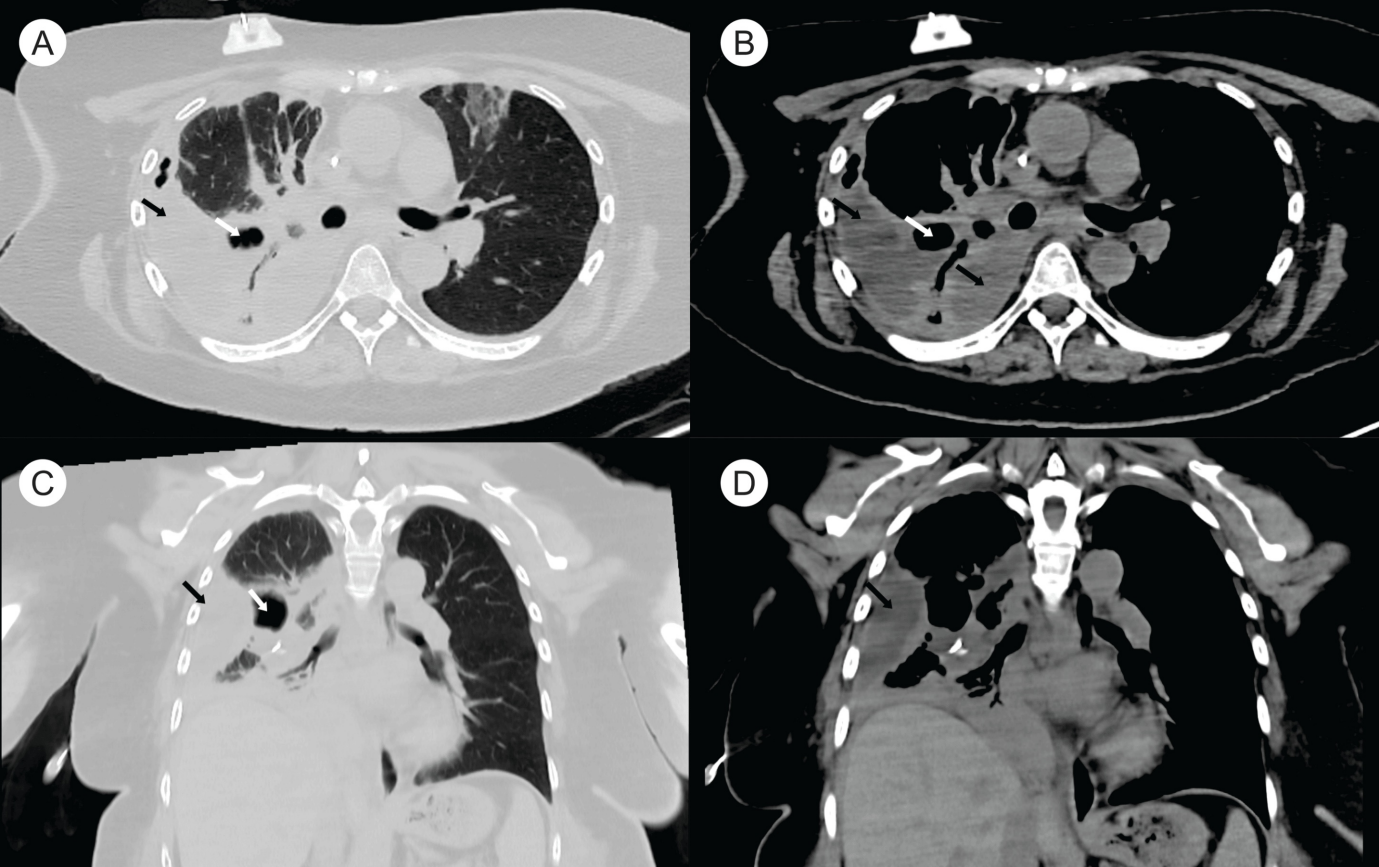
Chest CT after one week of treatment Chest CT after one week of chest drainage with a thoracostomy tube and empiric antibiotics showing incomplete resolution of the empyema (black arrows) and necrotic lung zones (white arrows). (A) Axial chest CT with lung window. (B) Axial chest CT with mediastinal window. (C) Coronal view with lung window. (D) Coronal view with mediastinal window.
